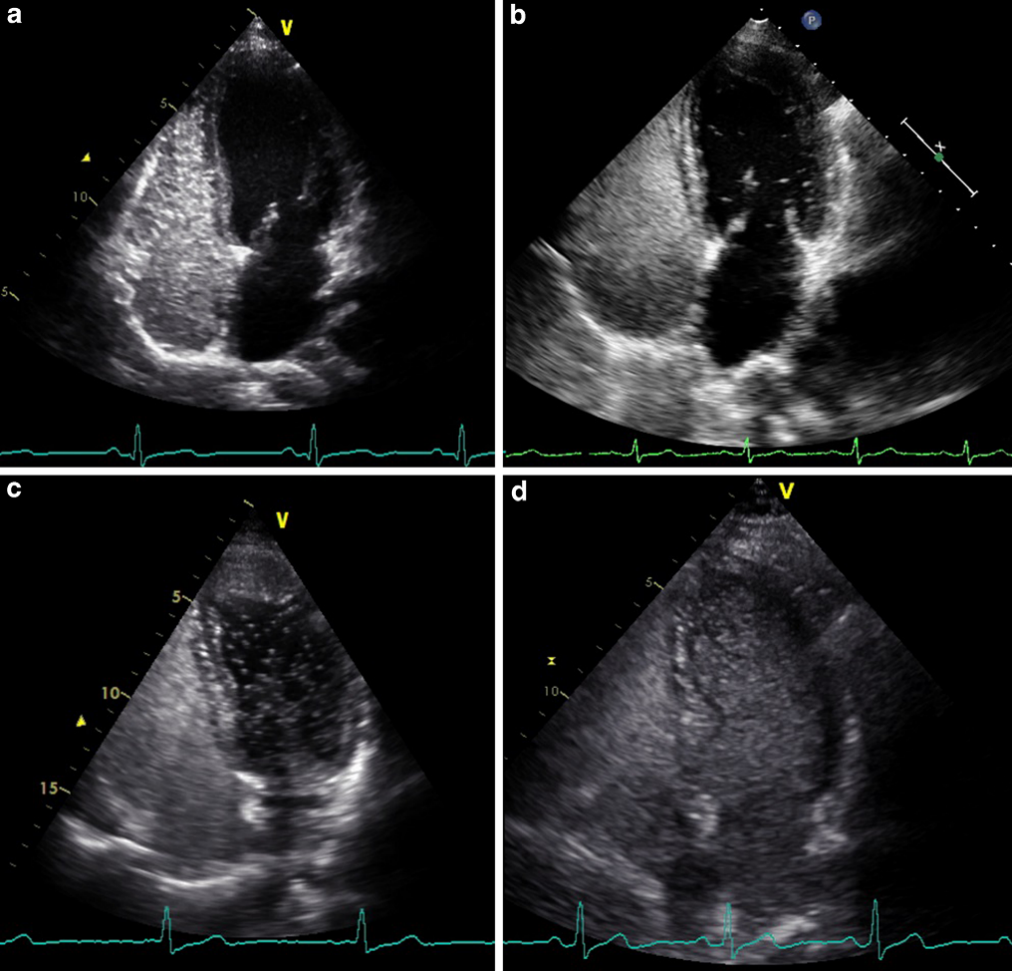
Pulmonary right-to-left shunt (RLS) on transthoracic contrast echocardiogram. a No pulmonary RLS. b Pulmonary RLS grade 1. c Pulmonary RLS grade 2. d Pulmonary RLS grade 3
